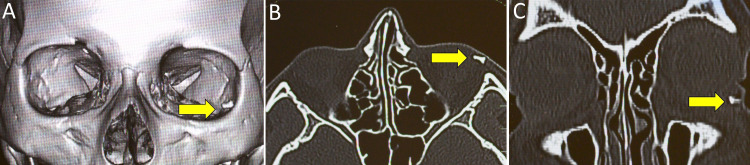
Three-dimensional reconstructed CT scan image showing the location (white arrow) of the foreign body with respect to the orbital rim (A). The sharp edges can be appreciated in the axial scan (B) and the coronal slice (C). CT: computed tomography
